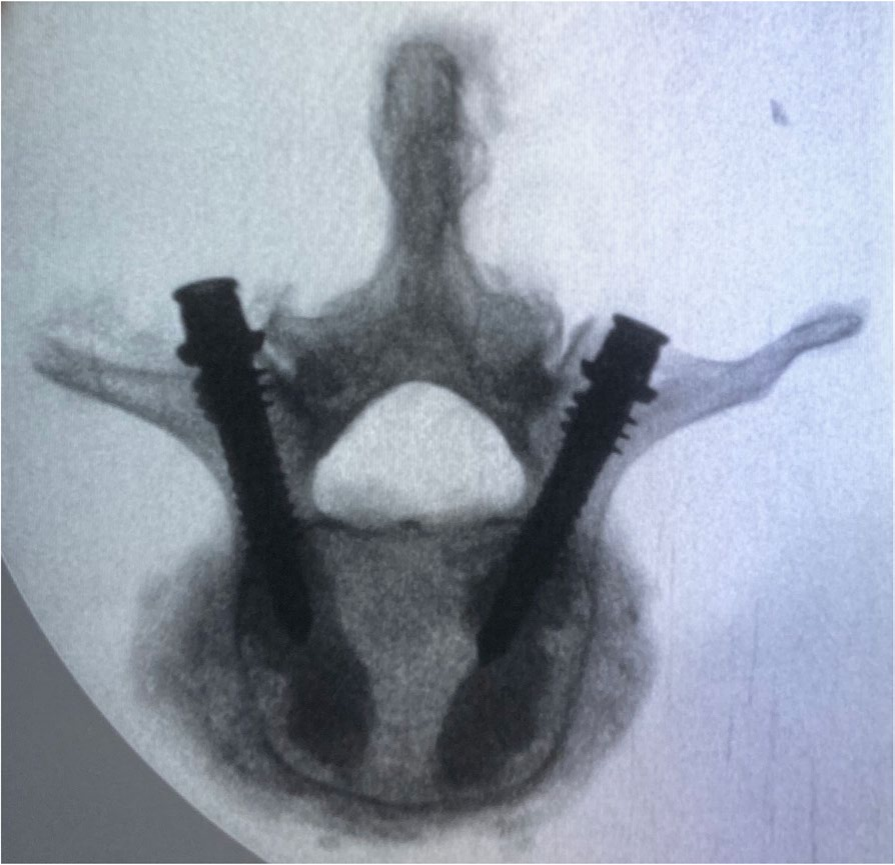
Example of radiograph after surgical treatment and biomechanical testing, which shows pedicle integrity
